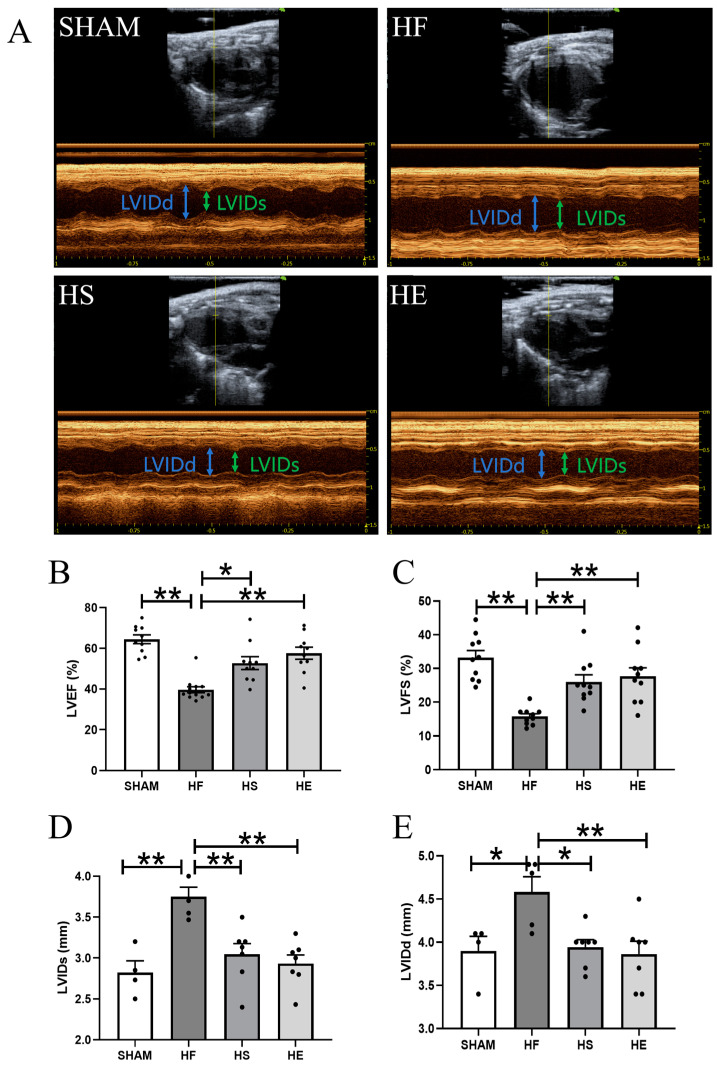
Aerobic exercise reduces TAC-induced cardiac dysfunction. (A) Representative images of cardiac ultrasound in each group; (B–E) were the quantitative analysis of left ventricular ejection fraction (LVEF), left ventricular short-axis shortening rate (LVFS), left ventricular internal diameter at end-systole (LVIDs), and left ventricular end-diastolic internal diameter (LVIDd) in each group. * indicates *p* < 0.05 compared with the HF group,  indicates *p* < 0.01 compared with the HF group.
